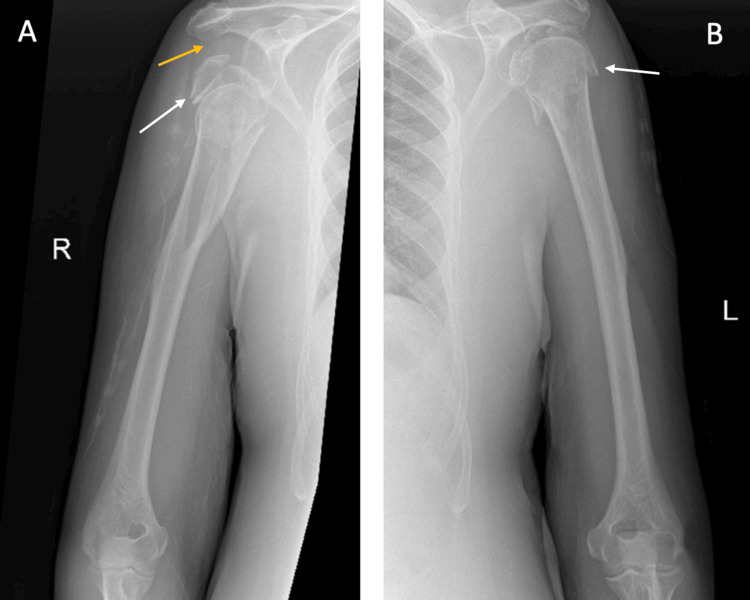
Pre-operative X-ray of the right (A) and left (B) shoulders and humeri. (A) Anteroposterior X-ray of the right shoulder including the right humeral shaft demonstrating a markedly displaced, angulated, and comminuted four-part proximal humerus fracture with significant inferior dislocation. (B) Anteroposterior X-ray of the left shoulder and left humerus showing displaced and comminuted four-part left proximal humeral head fracture with inferior subluxation of the humeral head. White arrows point to the fracture site. Yellow arrow shows the dislocation.
